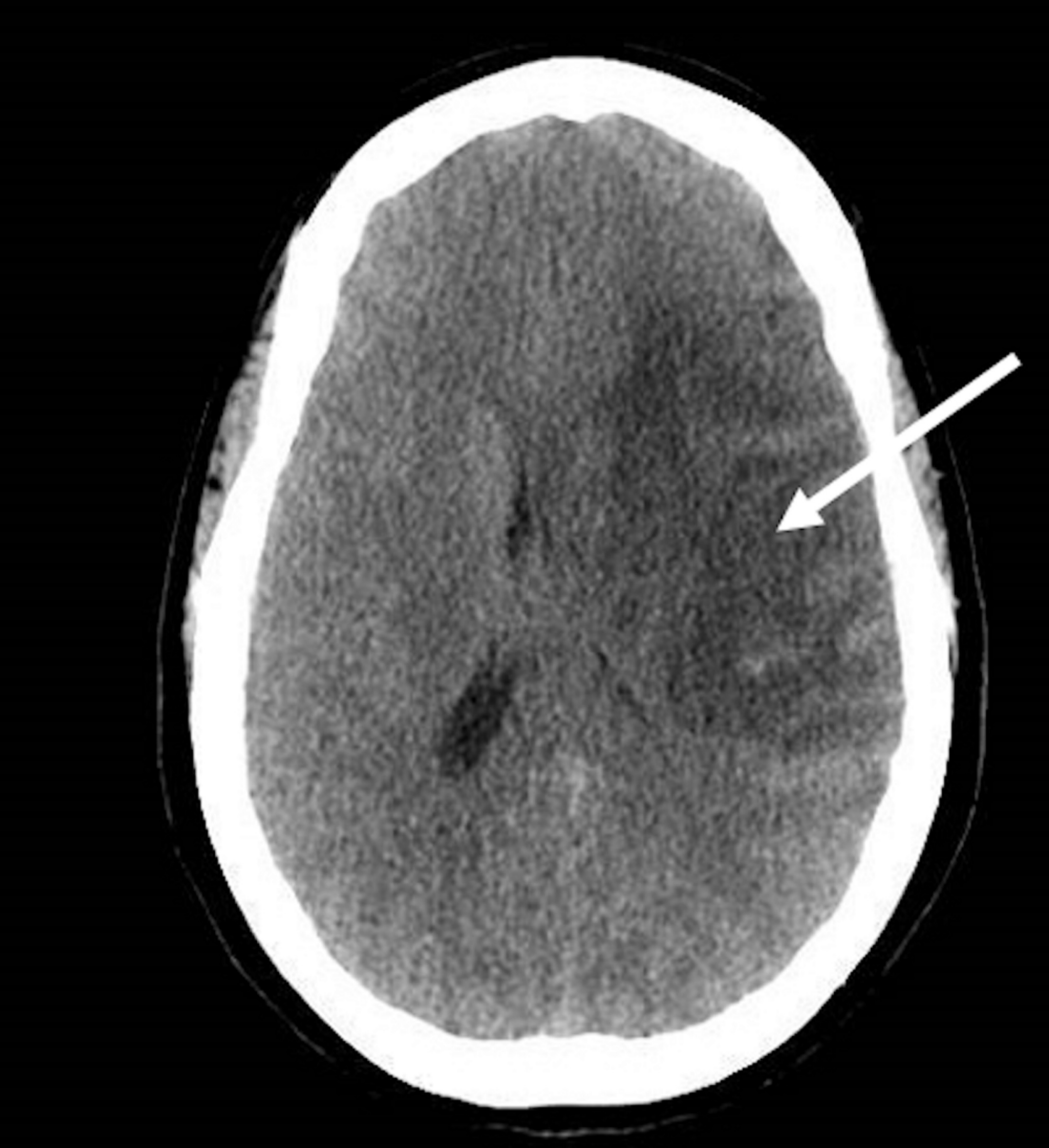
Computed tomography of the head showing a large acute on subacute infarction in the inferior portion of the left middle cerebral artery territory (white arrow).
